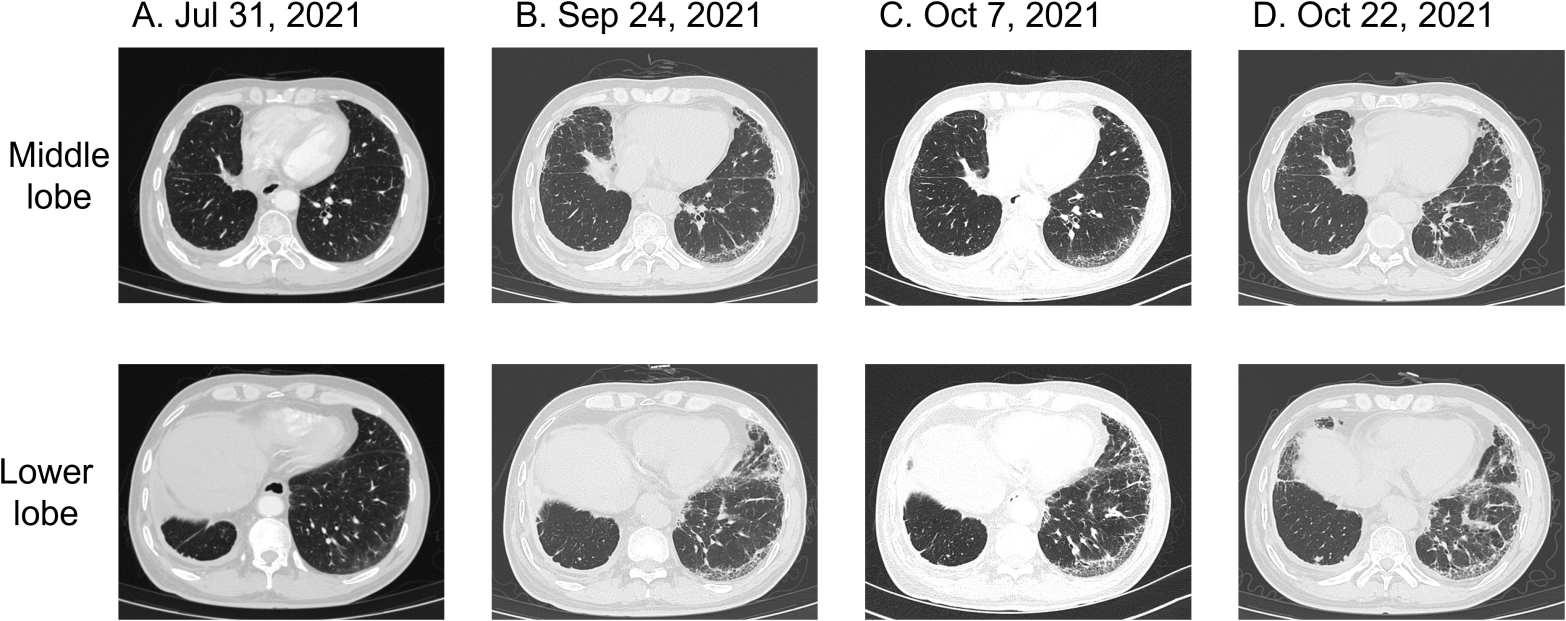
Pulmonary CT findings of interstitial pneumonia associated with cetuximab treatment for colorectal cancer. (A) Chest CT on July 31, 2021: mild interstitial changes in both lungs. (B) Chest CT on September 24, 2021: bilateral interstitial inflammation. (C) Chest CT on October 7, 2021: persistent interstitial fibrosis in both lungs. (D) Chest CT on October 22, 2021: mild progression of interstitial inflammation and localized fibrosis in both lungs, accompanied by pulmonary emphysema.
